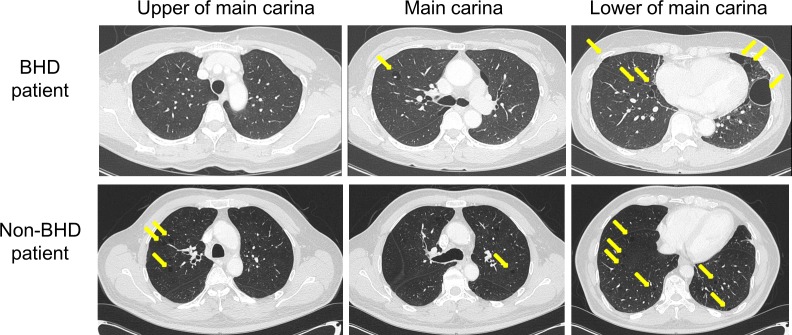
Chest CT image findings of pulmonary cysts *CT*, computed tomography; *BHD*, Birt–Hogg–Dube syndrome.
